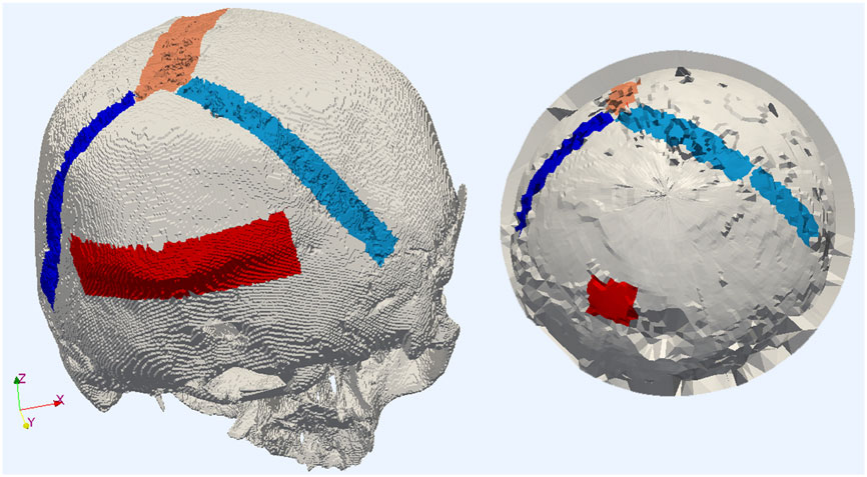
Datasets for surface registration. Left: 3D‐model from a segmentation of a CT‐image. Right: 3D‐model from an A‐mode ultrasound scan. The anatomical features in both models are marked with form attributes. The color differs for each suture of the Lambda fissure and the protuberantia occipitalis externa. (visualization: ParaView)
